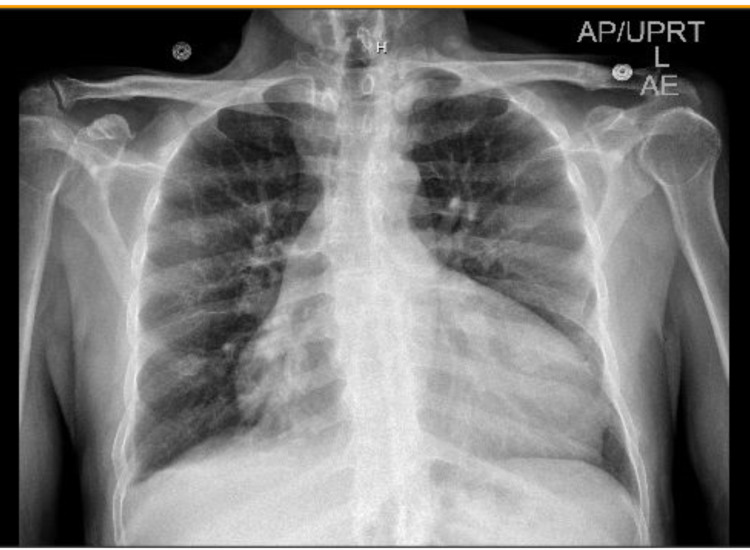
Standard posteroanterior chest X-ray CXR of a 49-year-old male who presented with a week of edema involving bilateral lower extremity, thighs, and scrotum, abdominal distention, cough productive of whitish phlegm, 18 pounds weight gain, orthopnea and dyspnea on exertion. The CXR shows enlarged cardiac silhouette, cardiomegaly, and pericardial effusion.
